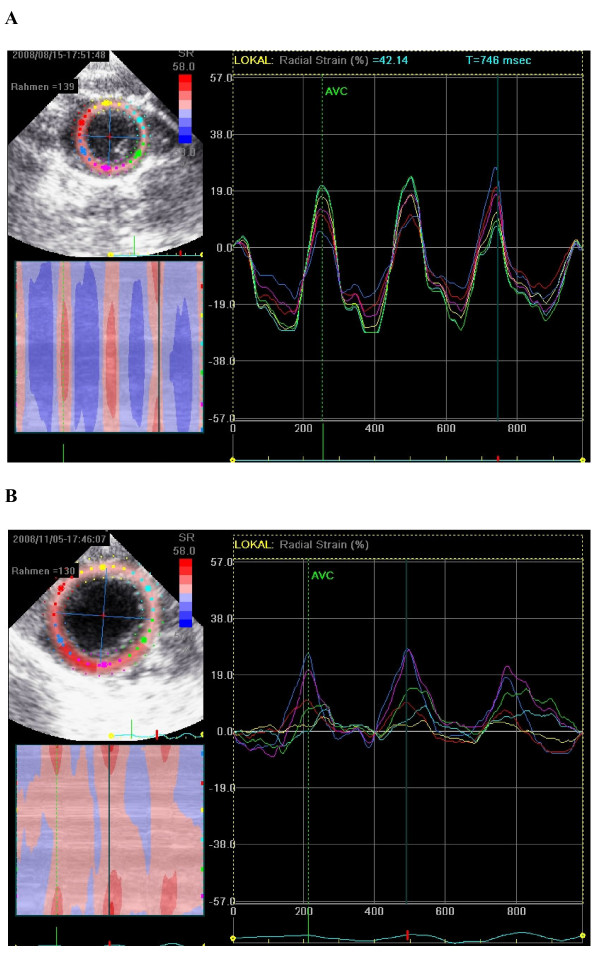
A and B: Non-Doppler radial 2D strain before (A) and after myocardial infarction (B). See flattened postoperative curves particularly septal (red), anteroseptal (yellow) and anterior (light blue).
